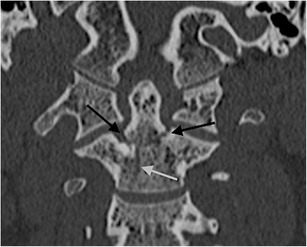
Complex type III odontoid fracture of the atlas in a 32-year-old male driver involved in a high-speed motor vehicle collision. Coronal reformatted CT image of the craniocervical junction demonstrates fracture extension into the body of the C2 vertebra (*black arrows*) with a further vertical fracture line extending through the right side of the body (*white arrow*)
